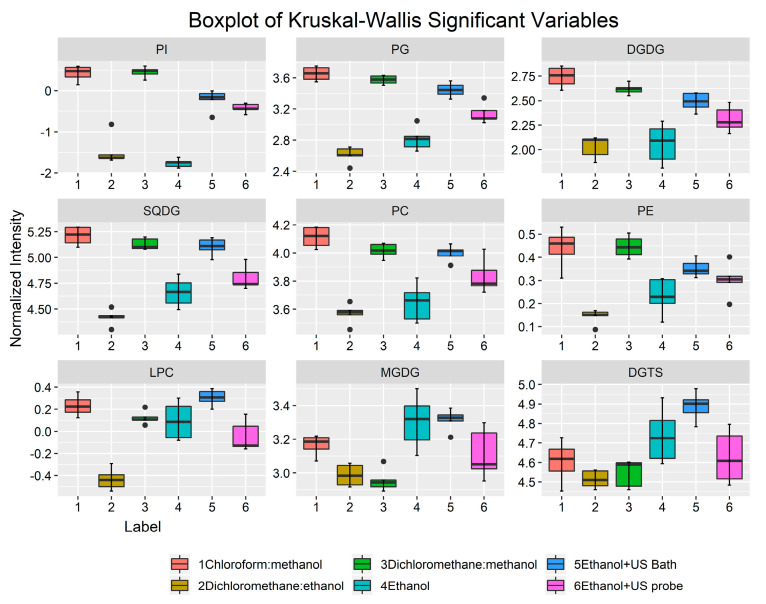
Boxplot of the polar lipid classes identified in lipid extracts of *Nannochloropsis oceanica* obtained using different lipid solvent extraction methods and ultrasound-assisted methodologies. The thick bar is the median value, and dots represent the outliers of five replicates. PC—phosphatidylcholine; LPC—lysophosphatidylcholine; PE—phosphatidylethanolamine; PI—phosphatidylinositol; PG—phosphatidylglycerol; DGDG—digalactosyl diacylglycerol; MGDG—monogalactosyl diacylglycerol; SQDG—sulfoquinovosyl diacylglycerol; DGTS—diacylglycerol-N,N,N-trimethyl homoserine.
